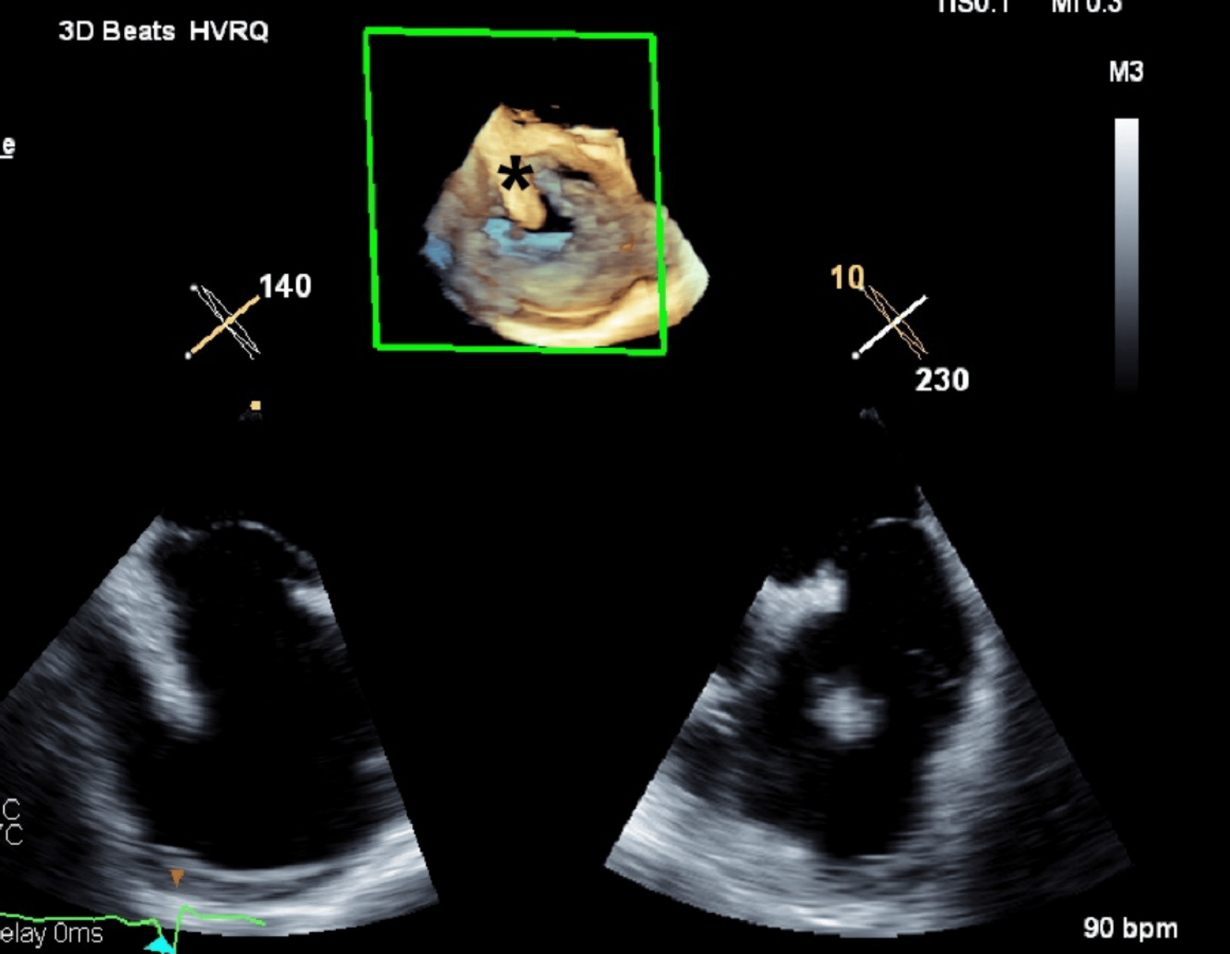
Transoesophageal echocardiogram cut showing the structure (asterisk) in 3D reconstruction 3D: three dimensional
